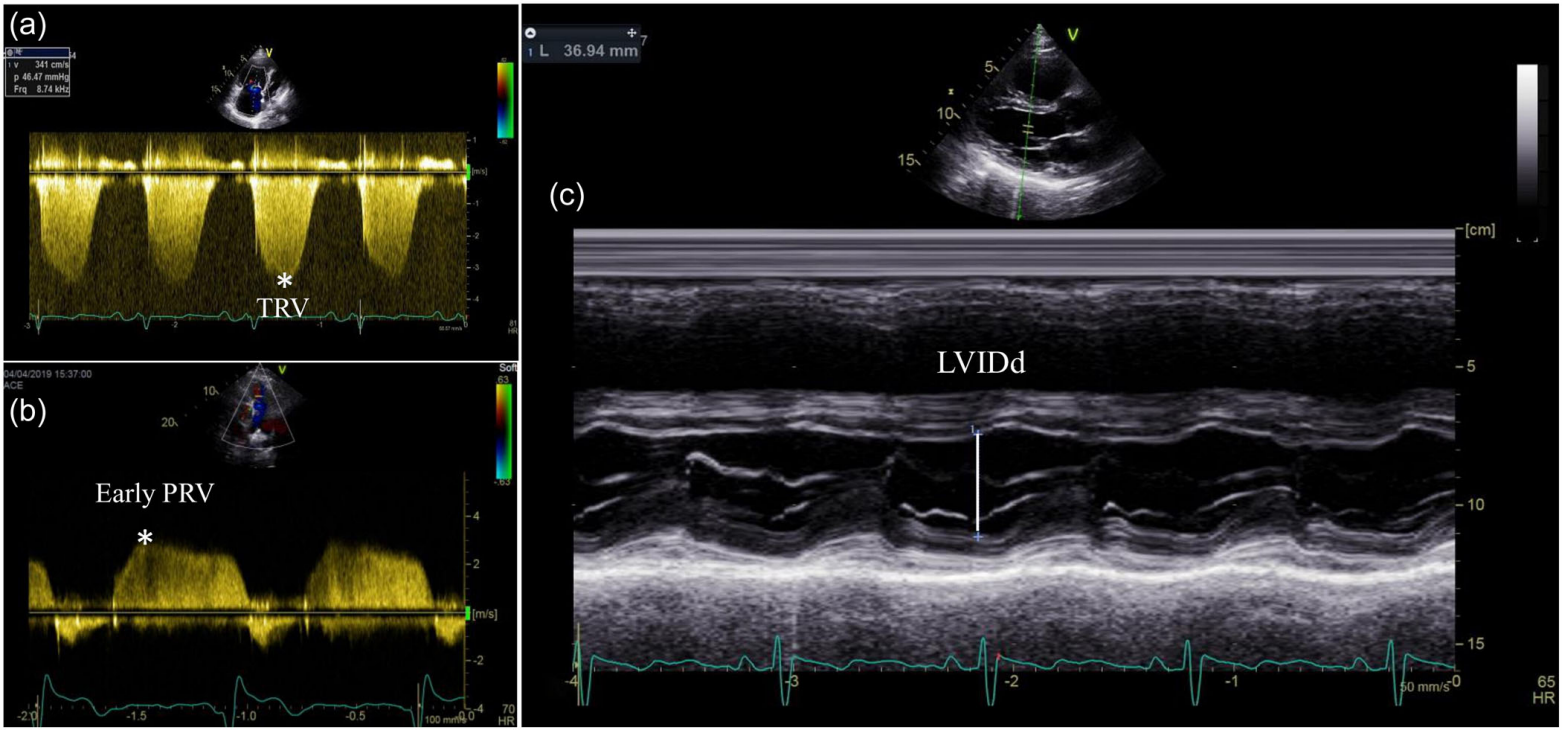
(a) The tricuspid regurgitation velocity (TRV) was measured to calculate systolic pulmonary artery pressure. (b) The early diastolic pulmonary regurgitation velocity (PRV) was measured to calculate mean pulmonary artery pressure. (c) Left ventricular internal diameter at end‐diastole (LVIDd) was acquired in the parasternal long‐axis view at a level of the mitral leaflet tips by M‐mode.
